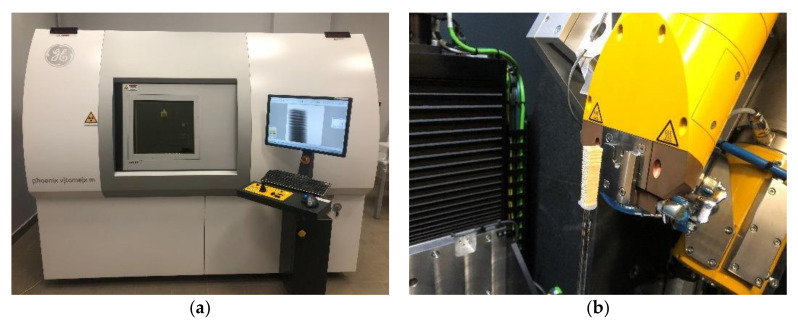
X-Ray CT studies. (a) GE Phoenix v-tome-x m device performing a test; (b) Sample prepared for a tomography test positioned inside the device.
